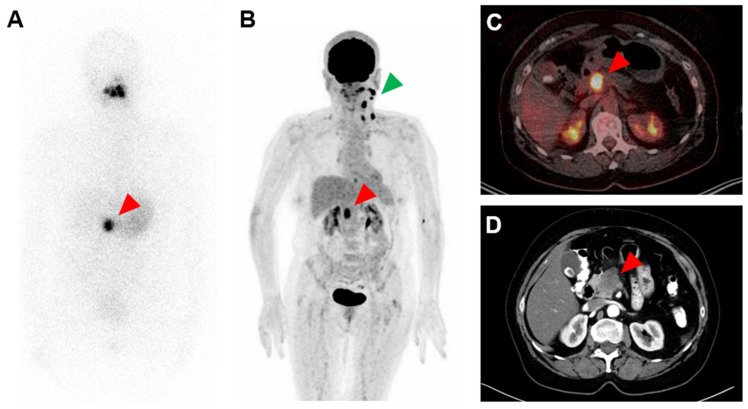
Strongly radioiodine (RAI)-positive and [^18^F]FDG-positive pancreatic adenocarcinoma. (A): ^131^I whole-body scintigraphy after administration of 3.8 GBq ^131^I, (B): MIP (maximum intensity projection) of [^18^F]FDG PET, (C): transversal slice of [^18^F]FDG PET/CT fusion and (D): transversal slice of contrast-enhanced CT. Red arrows point to RAI-positive and [^18^F]FDG-positive pancreatic adenocarcinoma; green arrow points to RAI-negative and [^18^F]FDG-positive lymph nodes metastases of papillary thyroid carcinoma in the left neck.
